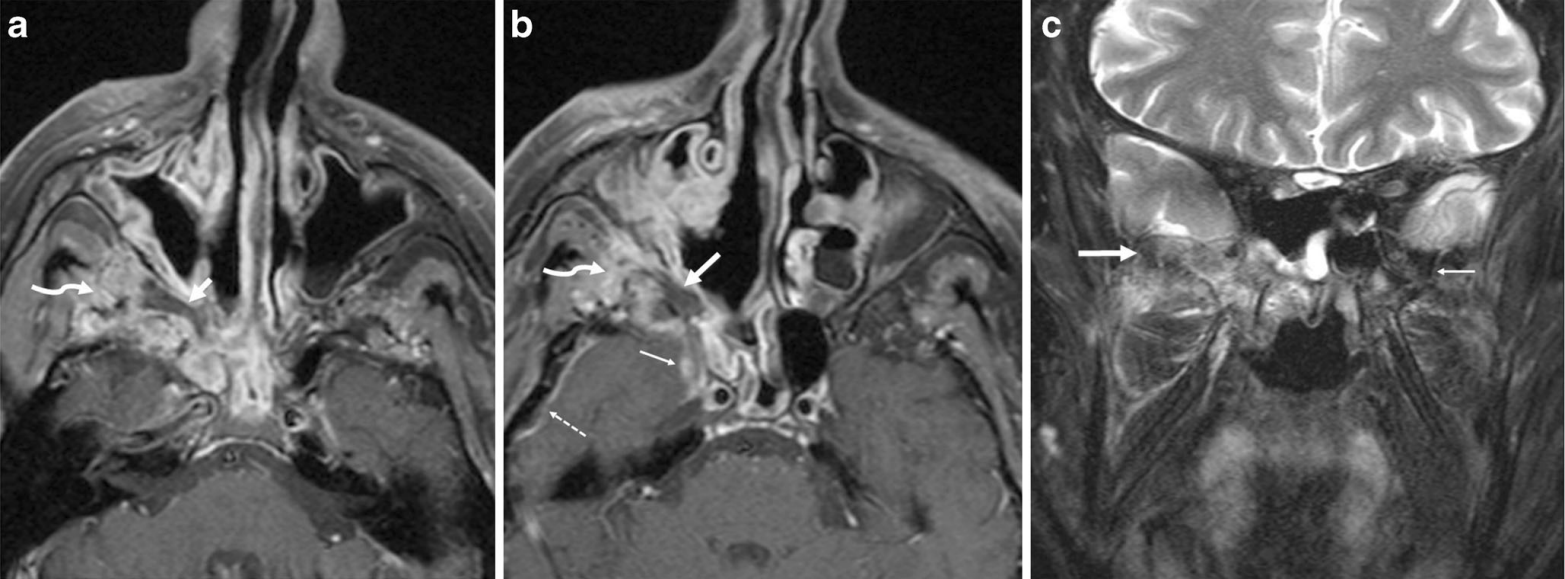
a Invasive fungal sinusitis caused by mucormycosis in a 54-year-old diabetic male. Axial contrast-enhanced FS T1W MR image shows right maxillary sinusitis with a formation of a rim-enhancing collection in the right PPF (*arrow*) in keeping with a fungal abscess (which was surgically drained later). Extension of the inflammatory changes into the right ITF (*curved arrow*) via the PMF. b Axial contrast-enhanced FS T1W MR image of the same patient shows involvement of the right V2 (*thin arrow*) and intracranial extension, as evidenced by the abnormal dural enhancement in the antero-lateral of the right middle cranial fossa (*dashed arrow*). The cranial aspect of the fungal abscess in the right PPF (*thick arrow*) with extension of the inflammation into the right ITF (*curved arrow*) and right sphenoid sinusitis. c Coronal inversion recovery MR image of the same patient shows marrow oedema of the right greater wing of the sphenoid bone (*thick arrow*) in keeping with fungal osteomyelitis. The left greater wing of the sphenoid bone (*thin arrow*) is normal
